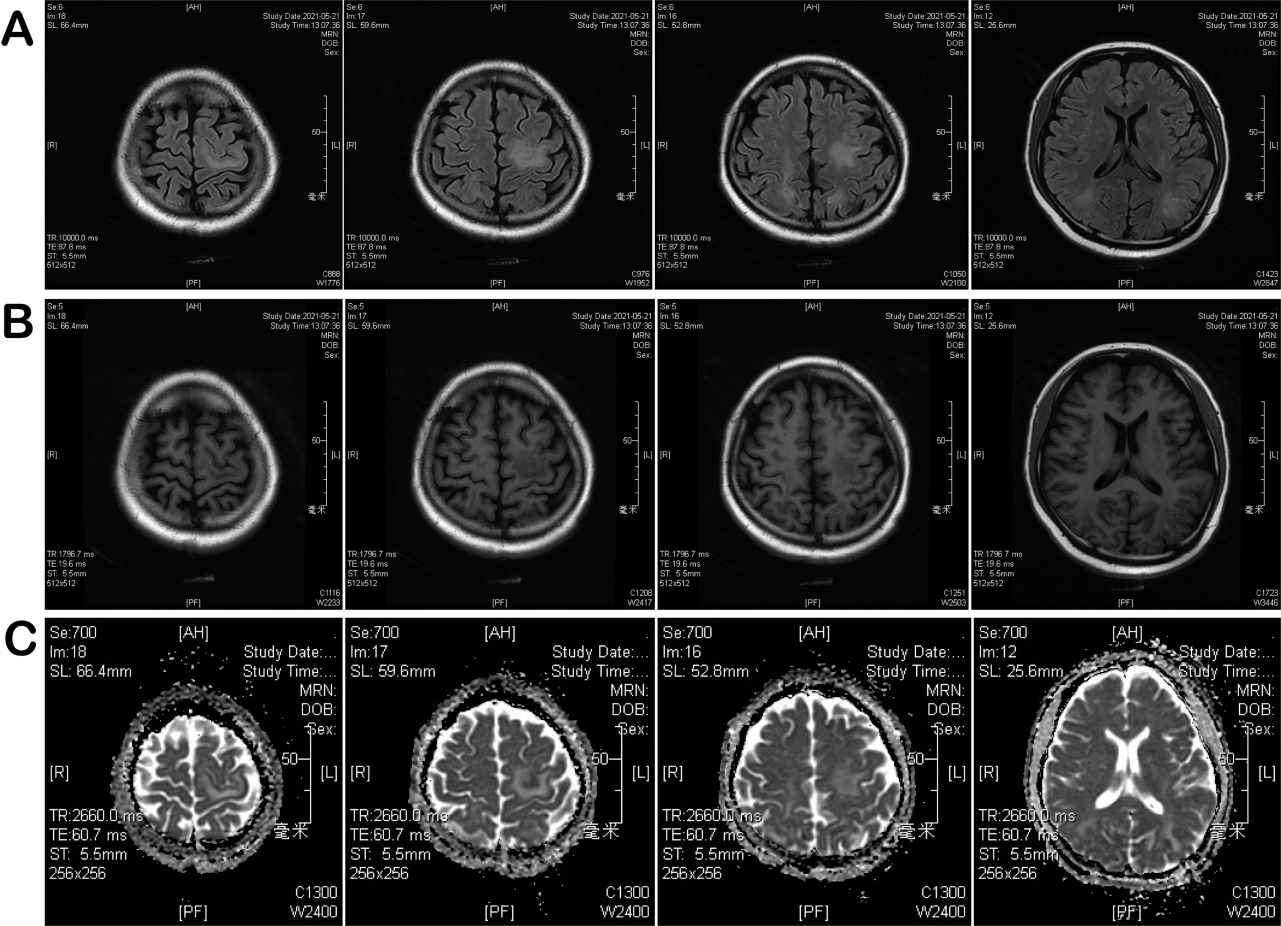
MRI performed on May 21, 2021: (A) Brain/spinal fluid-attenuated inversion recovery (FLAIR) sequence; (B) T1-weighted gadolinium-enhanced sequence; (C) diffusion-weighted imaging and apparent diffusion coefficient (DWI-ADC) maps.
